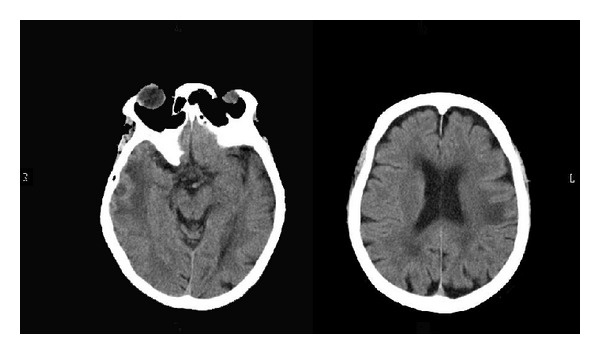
Brain CT performed during emergency care revealing a low density area with a nodular structure in the lower part of the right temporal lobe and a smaller low density area in the left paraventricular subcortical area suggestive of brain metastasis and brain edema.
